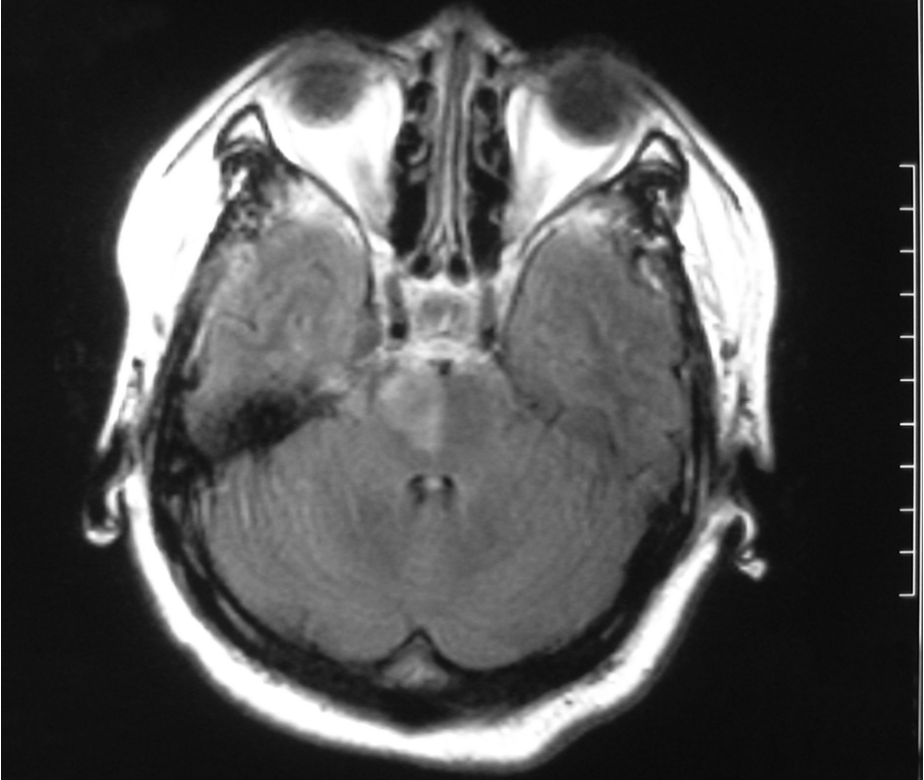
Magnetic resonance imaging performed 6 days after onset of the fixed hemiplegia. Axial fluid-attenuated inversion recovery (FLAIR) image shows well-delineated increased signal in the right aspect of the paramedian pontine.
